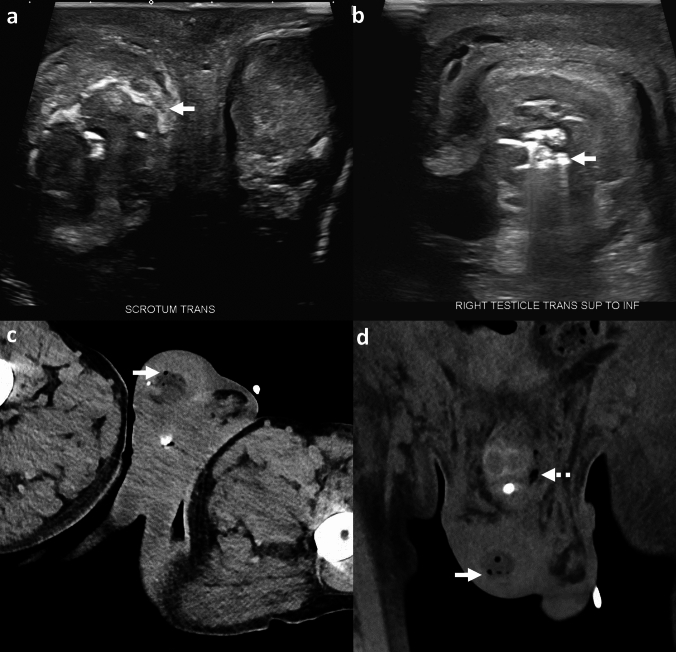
Emphysematous epididymo-orchitis. A 54-year-old with a foreign body insertion into the urethra, presenting with scrotal pain and difficulty voiding. Transverse US images show air within the enlarged right testis, seen as echogenic foci with dirty posterior acoustic shadowing (solid white arrow, a, b). CT images show air within the right testis (solid white arrows, c, d) with scrotal edema. Also note air in the penile shaft secondary to penetrating urethral injury (dotted white arrow, d). The patient underwent surgical debridement. Cultures showed *Escherichia coli*, *Proteus mirabilis*, *Streptococcus* species, and anaerobes
